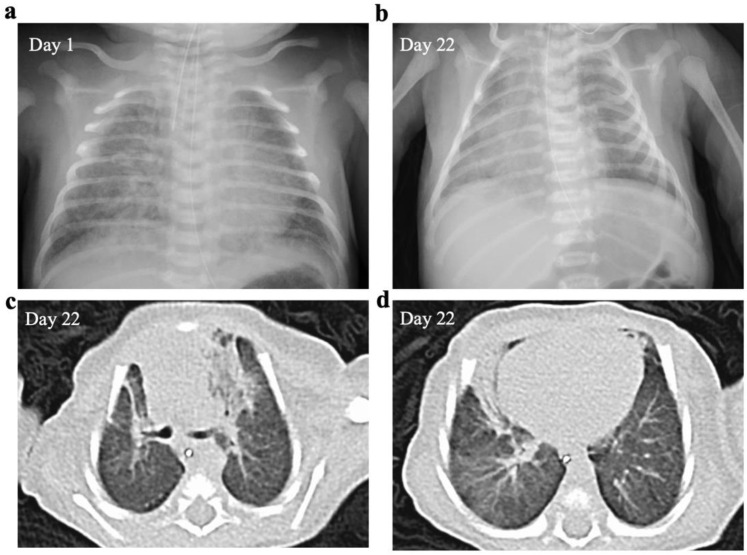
Chest radiograph and computed tomography (CT) scan. The chest radiograph displays bilateral hazy opacities (a). The chest radiograph (b) and CT examination (c,d) performed at 22 days of age reveal infiltrative shadows in both lung fields and decreased lung capacity.
